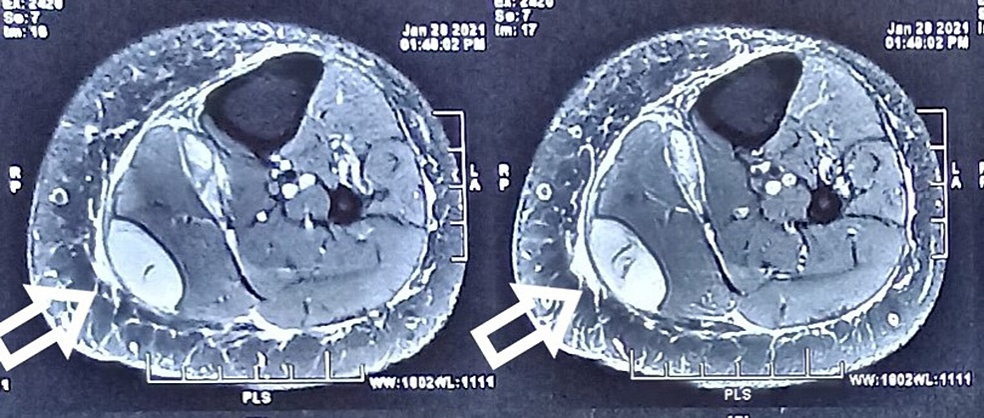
Axial T2-weighted image showing hyperintense cyst (arrows)
